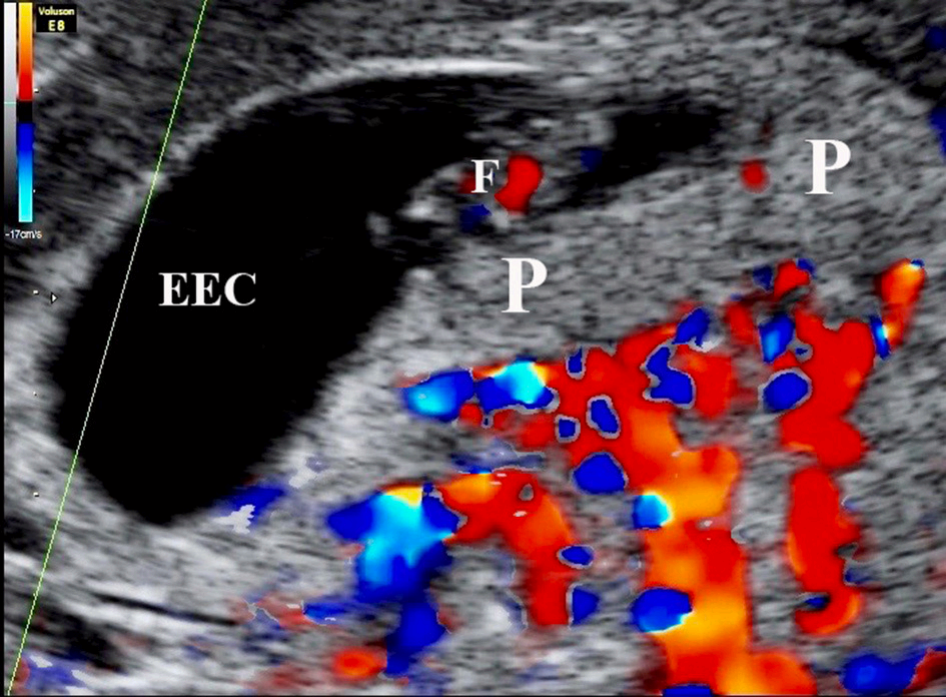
Color Doppler mapping of the utero-placental circulation at 7 weeks 2 days of gestation showing the absence of circulation within the placenta (P). ECC, exocoleomic cavity; F, Fetus.
